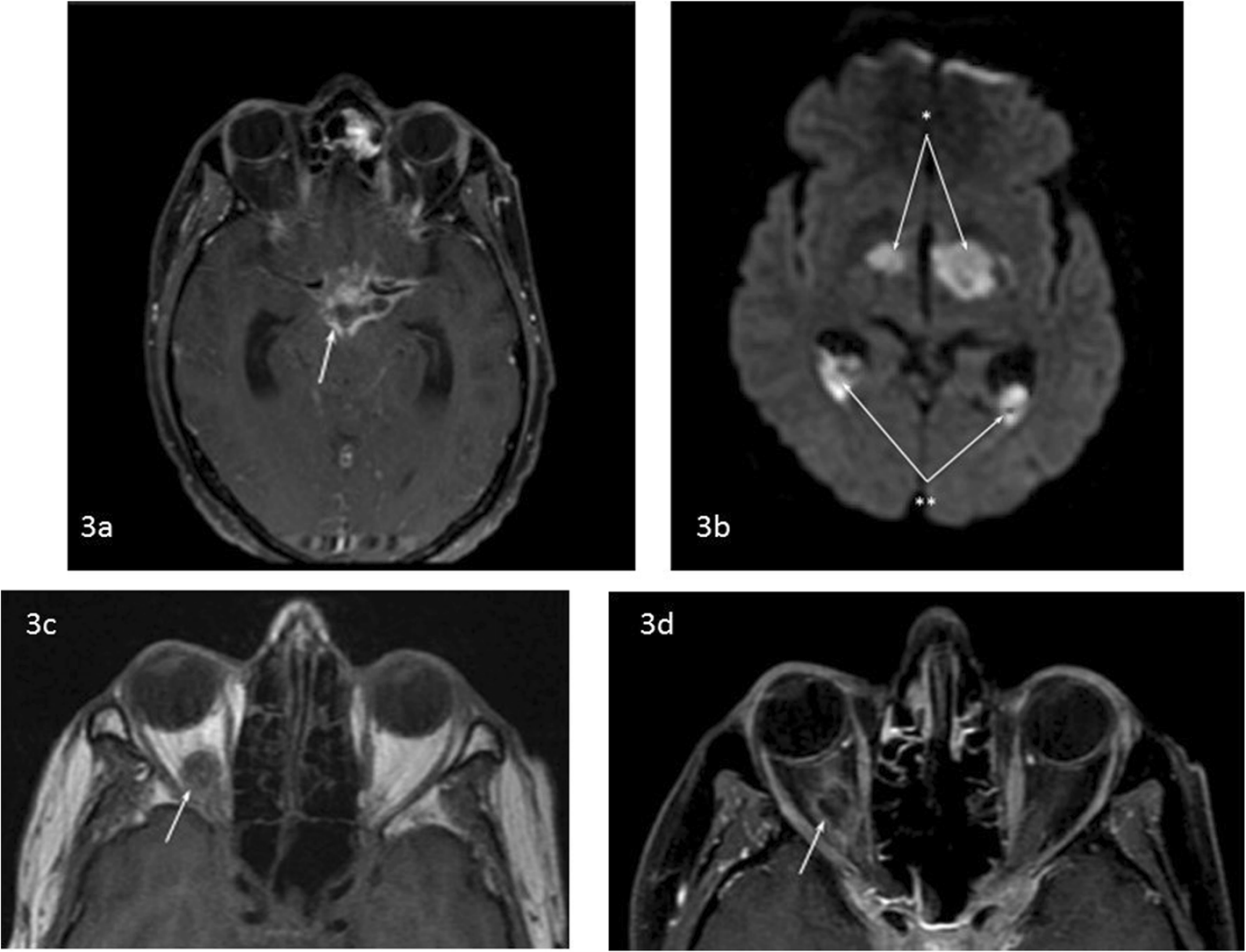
a T1w fat-suppressed post-gadolineum MRI scan which shows contrast enhancement at the surface of the basal brain structures compatible with basal meningitis (arrow). b Diffusion-weighted image shows high signal, which represents diffusion restriction caused by an acute brain infarct, bilaterally in the thalamus (arrow indicated by asterisk). In addition the high signal posterior in the ventricles is suggestive of ventricular empyema (arrow indicated by double asterisk). c and d Pre and post-gadolineum T1w MRI scan demonstrating a lesion in the right orbit with ring enhancement compatible with an abscess
